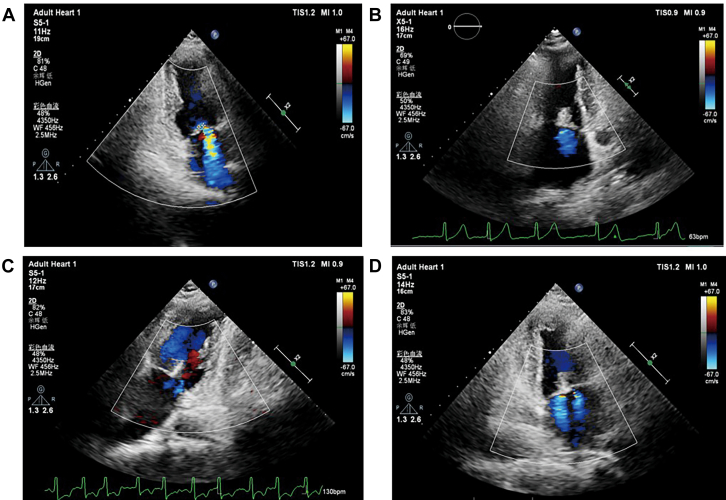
Transthoracic Echocardiography Images Obtained During Follow-Up (A) Before transcatheter mitral valve edge-to-edge repair (TEER). (B) Postoperative day 2. (C) One month after TEER. (D) Four months after TEER.
